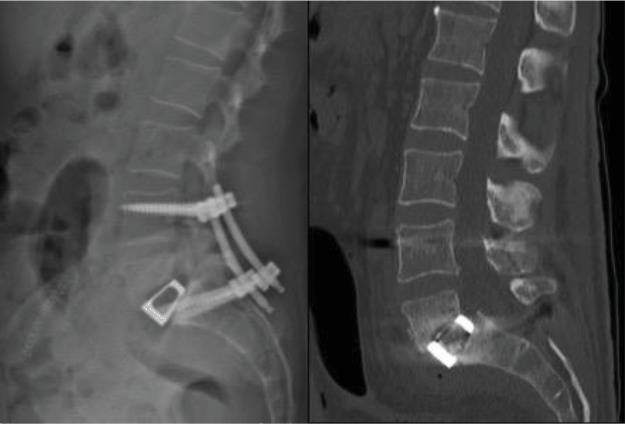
Postoperative (a) lumbar spine radiography in standing position and (b) lumbar CT scan, demonstrating the restoration of the lumbar lordosis and partial reduction of the L5-S1 spondylolisthesis.
